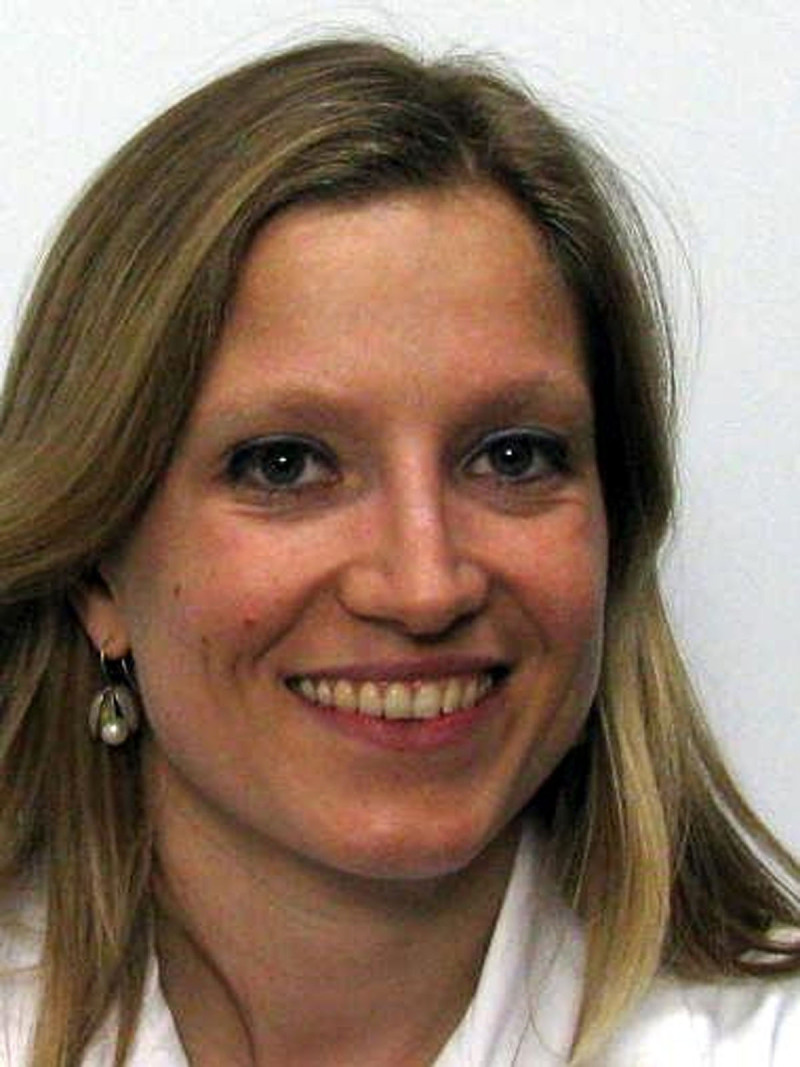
Prof. Dr. Raphaëla (Elleke) Dresen (MD, PhD) works as an abdominal radiologist at the University Hospitals Leuven since 2014. She obtained her medical degree from the University of Maastricht, the Netherlands, in 2006 and earned her PhD at the Faculty of Health, Medicine and Life Sciences in Maastricht in 2009. Her dissertation was addressed to the multidisciplinary approach to locally advanced and recurrent rectal cancer. She practices all aspects of abdominal radiology, including magnetic resonance imaging (MRI), computed tomography (CT), positon emission tomography coupled with CT (PET/CT), ultrasound, fluoroscopy and nonvascular abdominal interventions. She is an active member of the oncological multidisciplinary team meetings, especially concerning gastro-intestinal, colorectal, and gynaecological cancer patients. Since October 2017 she is partly employed as an assistant professor at KU Leuven. She is involved in the education of students, interns and residents. Her current research addresses oncological imaging, with a special focus on rectal cancer imaging and whole-body diffusion-weighted magnetic resonance imaging in patients with gastro-intestinal, colorectal, and gynaecological cancer with the aim of optimization of treatment planning and assessment of treatment response. She is a faculty member of the biannual ESGAR workshop “MR imaging of rectal cancer”.
